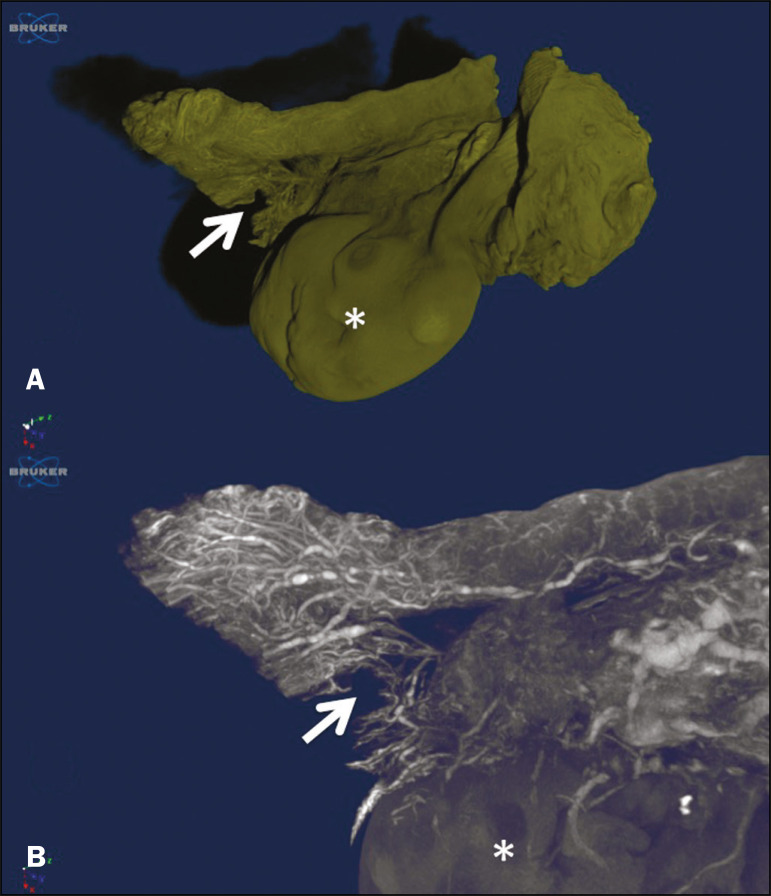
Left FT and ovary from a hysterectomy and salpingo-oophorectomy. A: Surface reconstruction of the adnexa, demonstrating part of the myometrium on the right. The ovary was normal (asterisk), and the arrow shows the ovarian fimbria. B: CT image of the left adnexa. Note the intense vascularization of the fimbriae compared with the other segments of the tube and the vascular connection from the ovary (asterisk) to the fimbriae (arrow, ovarian fimbria).
